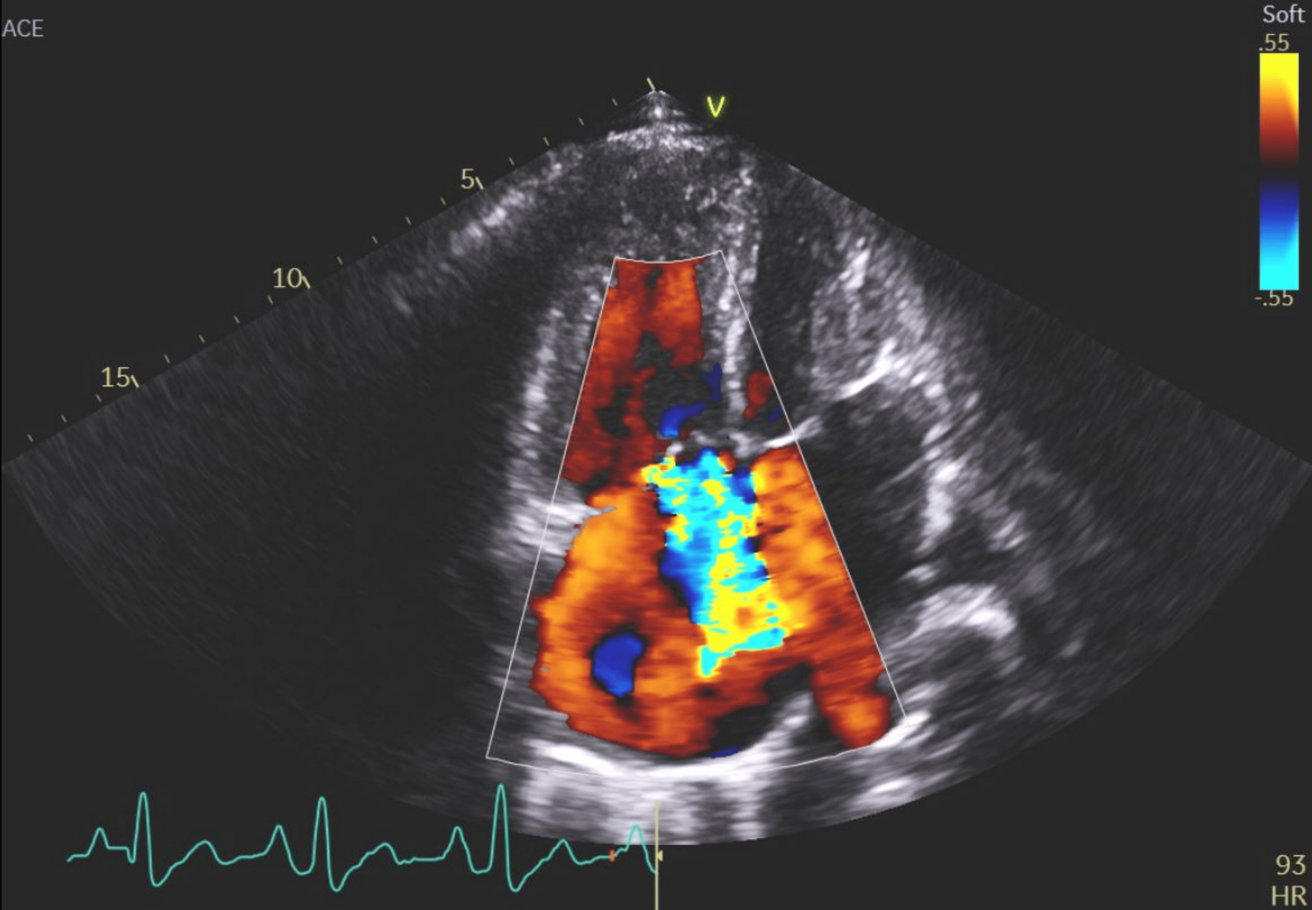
An echocardiographic image in the apical four-chamber view with color Doppler showing a left-to-right leak through the atrial septum.
